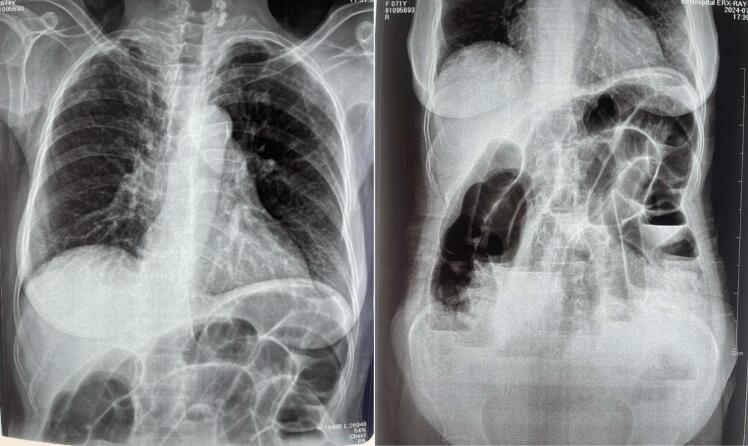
X-ray chest and abdomen erect view showing dilated bowel loop with multiple air-fluid levels suggesting intestinal obstruction.
